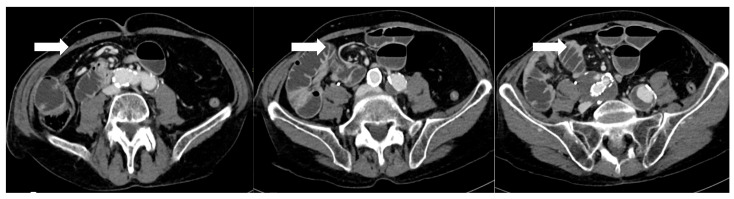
After 5 days from sleeve gastrectomy, axial post-contrast CT scans showing an angulated course of the duodenojejunal junction to the right of the midline, with evidence of a mesenteric “whirlpool sign,” suggestive of intestinal malrotation (arrows). The copyright of this figure belongs to the authors.
